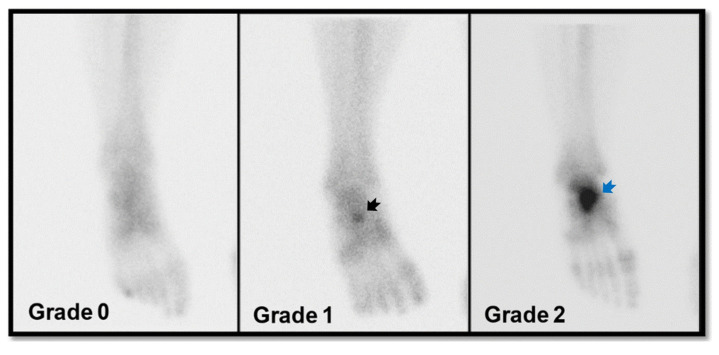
Visual grading criteria for Tc-99m MDP uptake in the navicular bone on conventional planar image of Tc-99m MDP bone scintigraphy. Grade 0: no visible focal uptake in the navicular region; Grade 1: mildly increased uptake compared with adjacent tarsal bones (black arrow); Grade 2: markedly increased focal uptake clearly exceeding the intensity of surrounding osseous structures (blue arrow).
